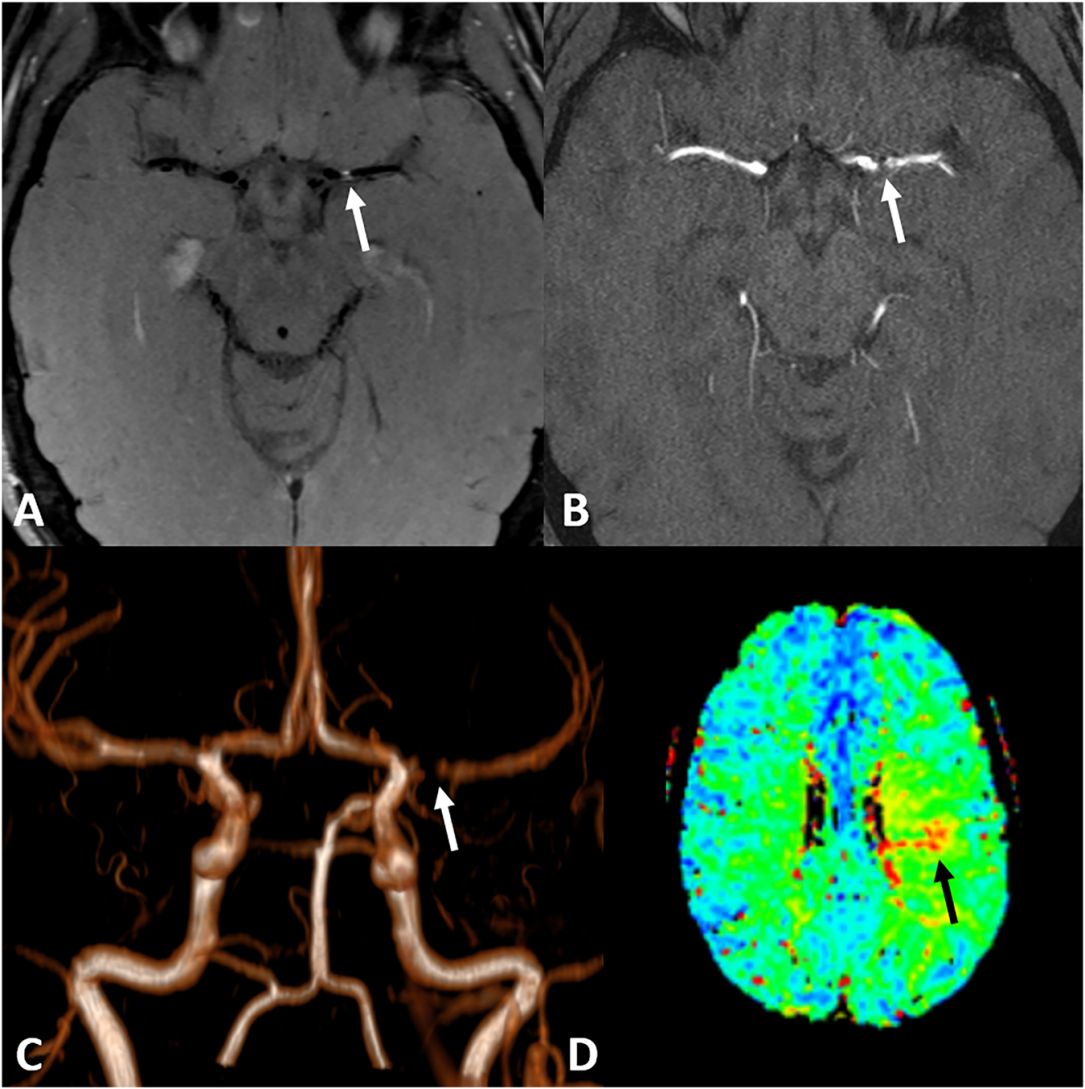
Initial imaging of a 29-year-old male patient, presenting with transient motoric aphasia. The proximal, left MCA shows focal vessel wall enhancement on axial T1 TSE dark blood (arrow) (A) with stenosis seen on TOF (B, C) (arrow) with reduced perfusion on time to peak (arrow) (D). No infarction was seen on DWI. Immunosuppressive therapy was subsequently initiated
